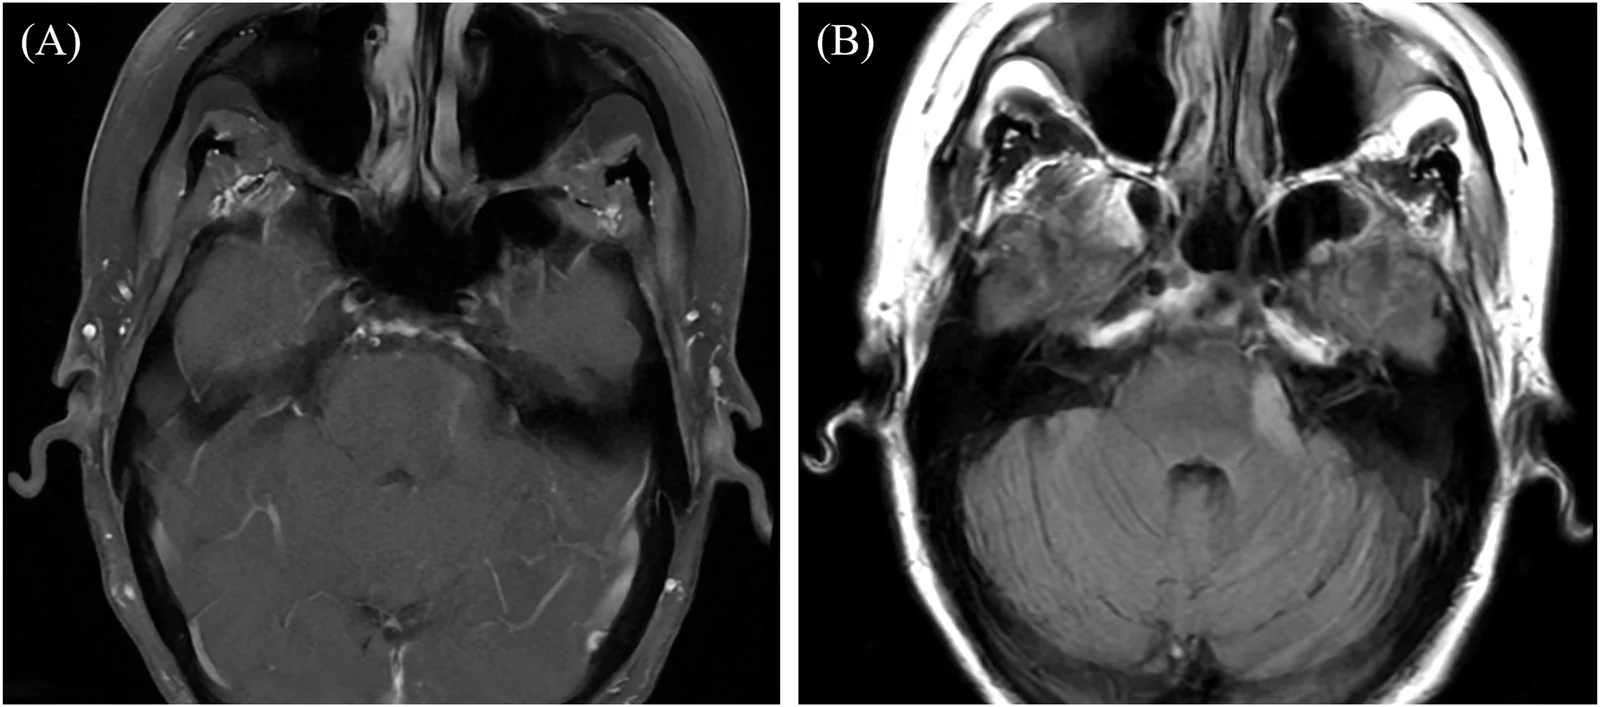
Magnetic resonance image with contrast at 10-month follow-up (A) T1-post and (B) T2- Fluid-Attenuated Inversion Recovery diminished signal in the lateral pons on the left side of the root entry zone after 3 doses of bevacicumab with no improvement in symptoms.
